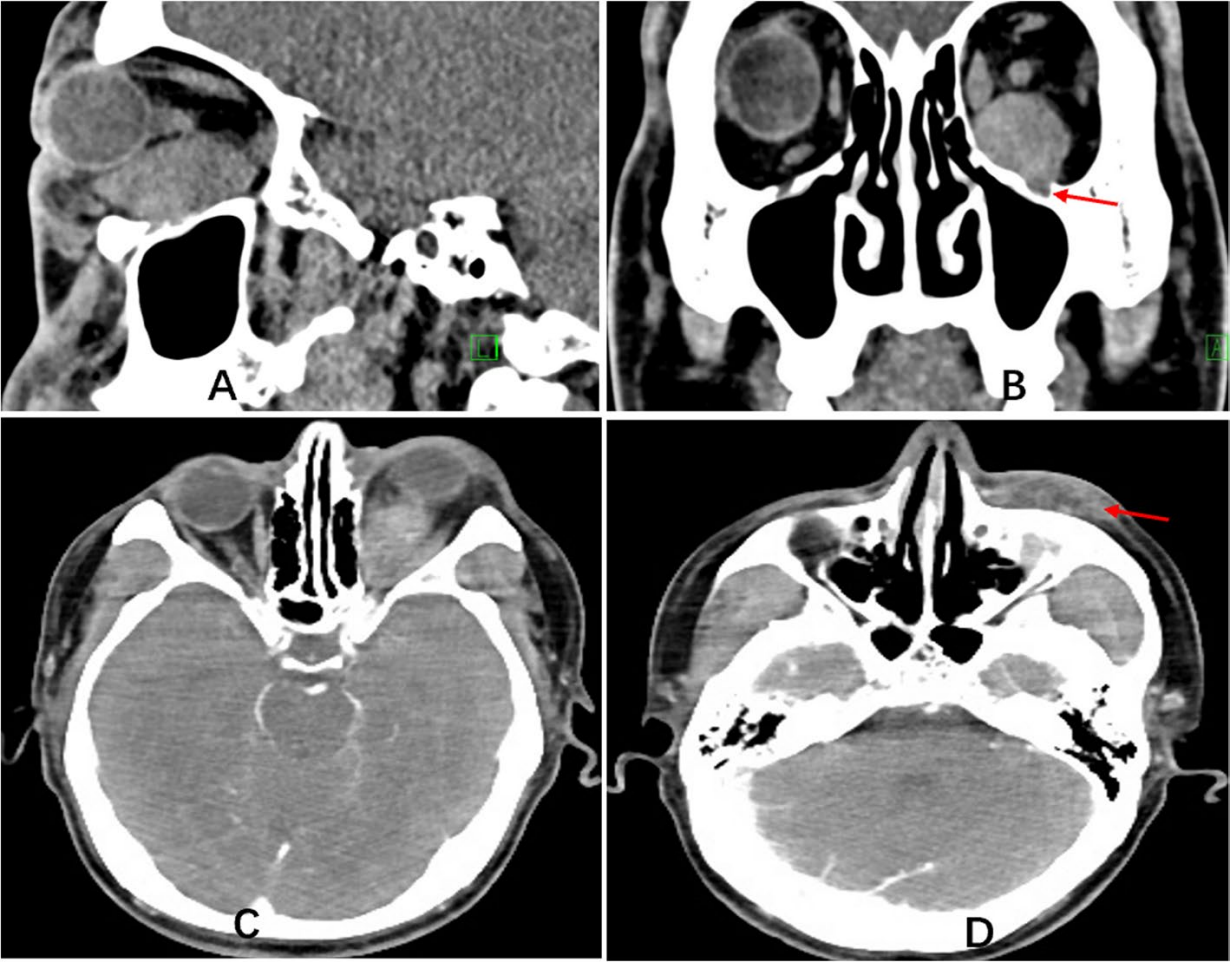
Orbital CT. A shows the lesion mass is medium density. The deep part is adhered to the optic nerve (sagittal scan); B shows part lesion invades the infraorbital fissure (coronal scan); C shows the lesion is adjacent to the medial rectus muscle and is inhomogeneous contrast enhancement (horizontal enhanced scan); D reveals the subcutaneous thickening (horizontal enhanced scan)
